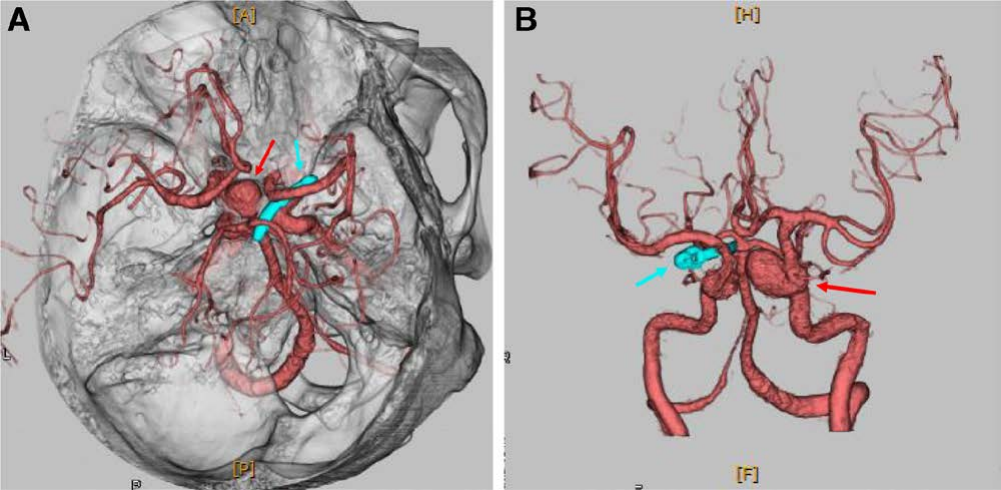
Three-dimensional computed tomography of the brain. The blue arrow indicates the clip for the prior cerebral aneurysm, whereas the red arrow indicates a new 1.8-cm aneurysm in the contralateral extracranial internal carotid artery.
